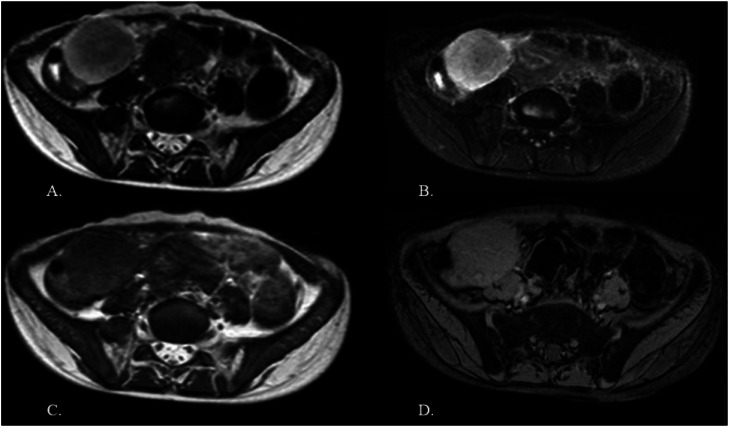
(A and B) T2-weighted and T2-SPAIR images show a well-defined, hyperintense lesion in the right iliac fossa. (C) The T1-weighted image shows the lesion is isointense. (D) No evidence of blooming is noted on the gradient echo sequence.
